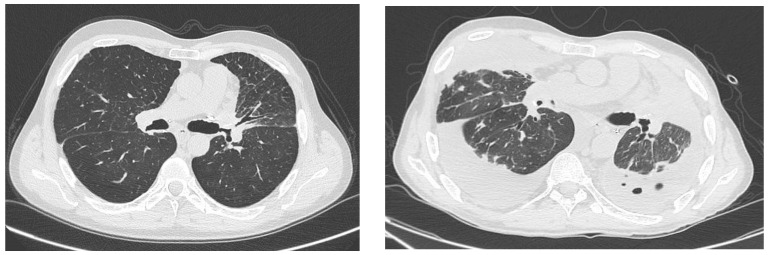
Case #2. Chest CT in March 2021 (left) and February 2023 (right). The lung parenchymal involvement worsened in February 2023, compared with March 2021, with the development of pneumonia and pleural effusion.
